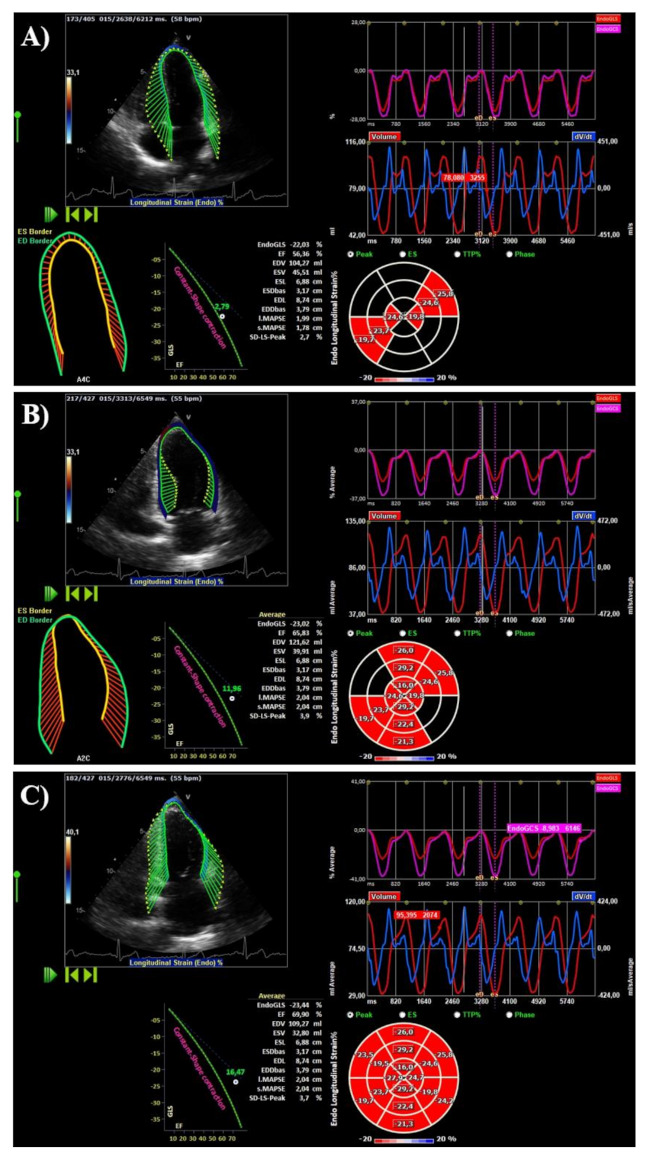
Post processing steps. (A) Analysis of apical 4 chamber view with vector velocity imaging, representation of endo GCS and GLS, variation of volumes and dV/dt, segmental longitudinal strain showed as bull-eye. (B) Analysis of 2 chamber view. (C) Analysis of 3 chamber view with average data. GCS, global circumferential strain; GLS, global longitudinal strain.
